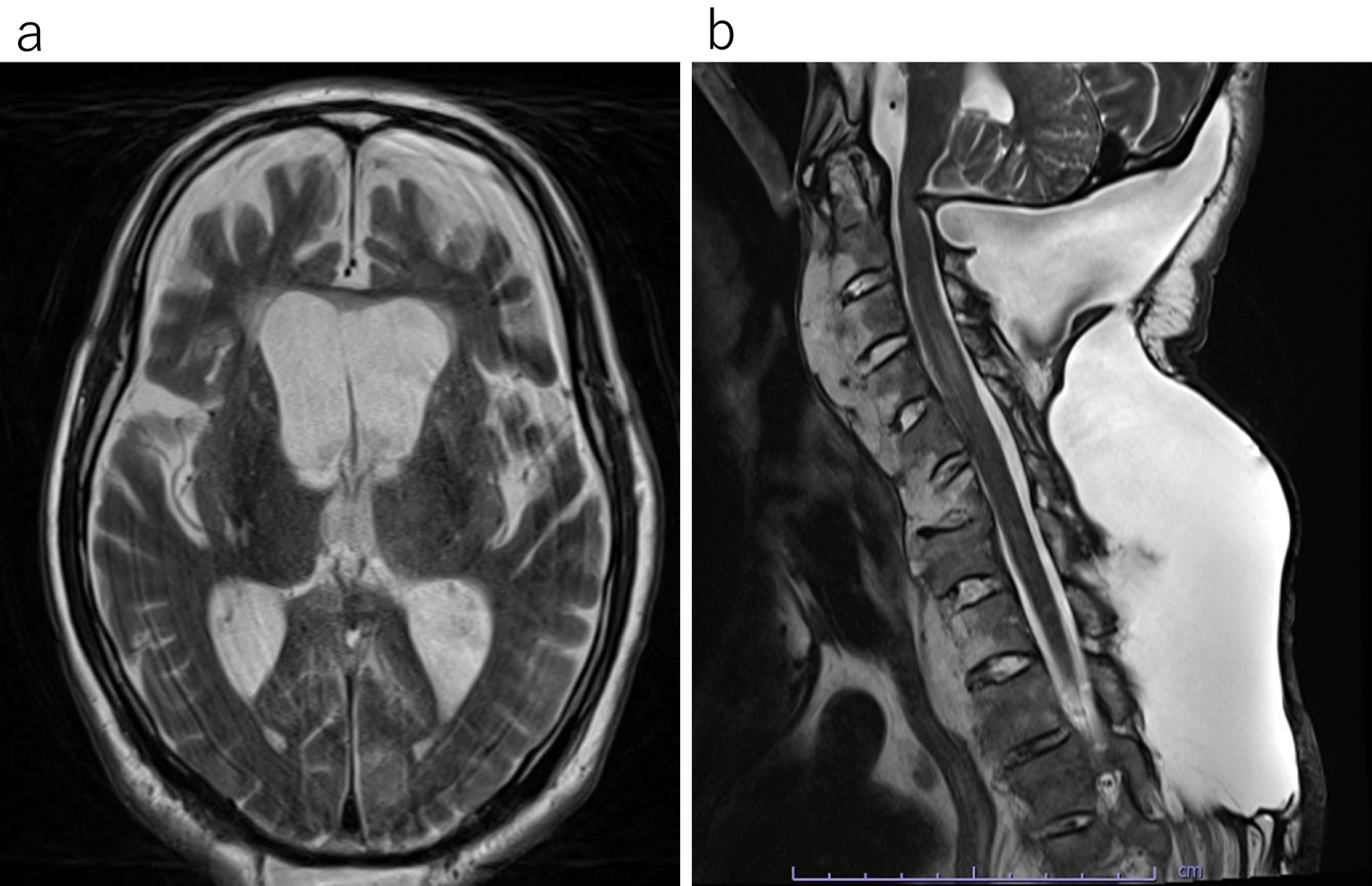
Four months postsurgery, the patient exhibited drowsiness and vomiting. Head and cervical MRIs were performed in response to these symptoms. The head MRI revealed considerable ventricular enlargement (a), whereas the cervical MRI indicated an increase in the pseudomeningocele (b).
